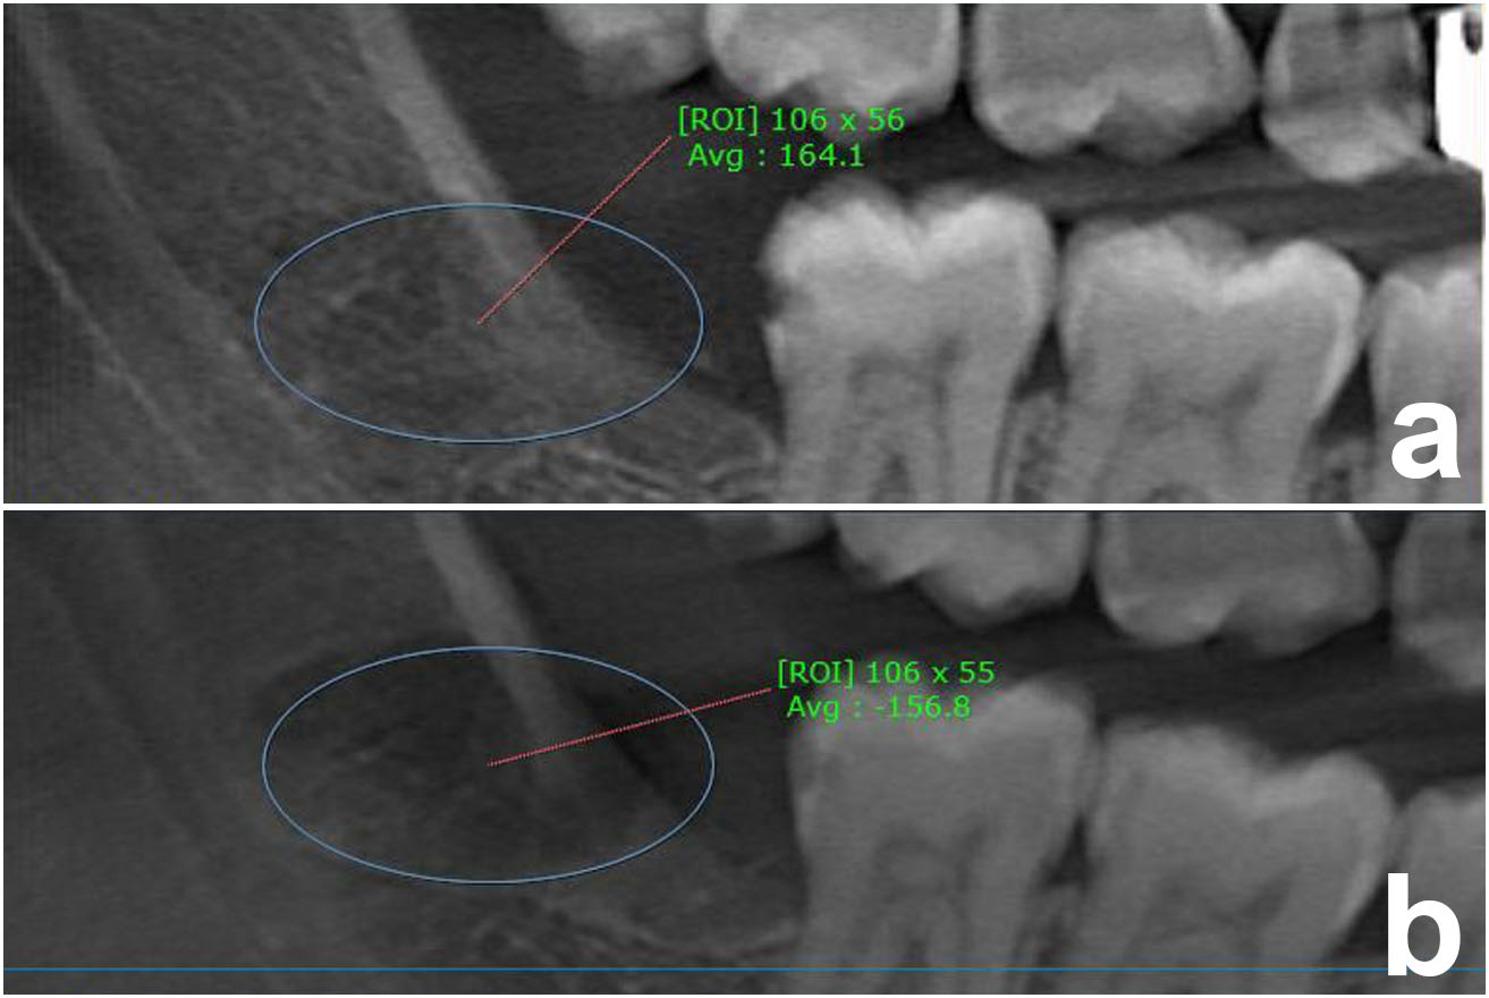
CBCT images demonstrating bone density assessment at the extraction site using a defined region of interest (ROI). a Representative CBCT slice showing the selected ROI within the extraction socket with a mean gray value of 164.1; b CBCT slice demonstrating ROI-based bone density measurement with a mean gray value of 156.8
